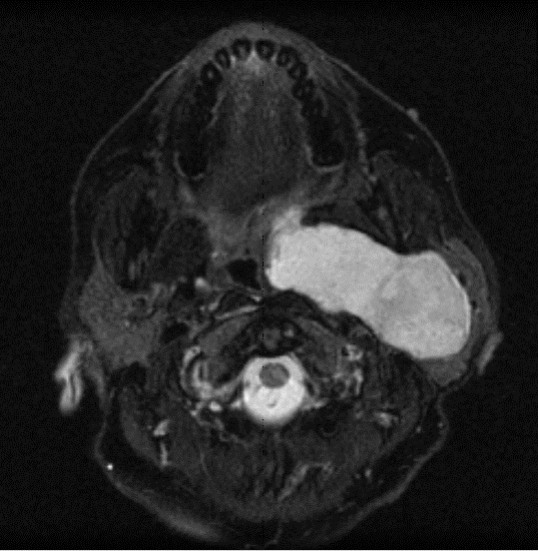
An axial T2-weighted MRI of the head and neck region (36th week of pregnancy). The outline of the heterogeneous left parotid lesion is again clearly demarcated and tissue planes preserved. The oropharyngeal lumen is distorted.
